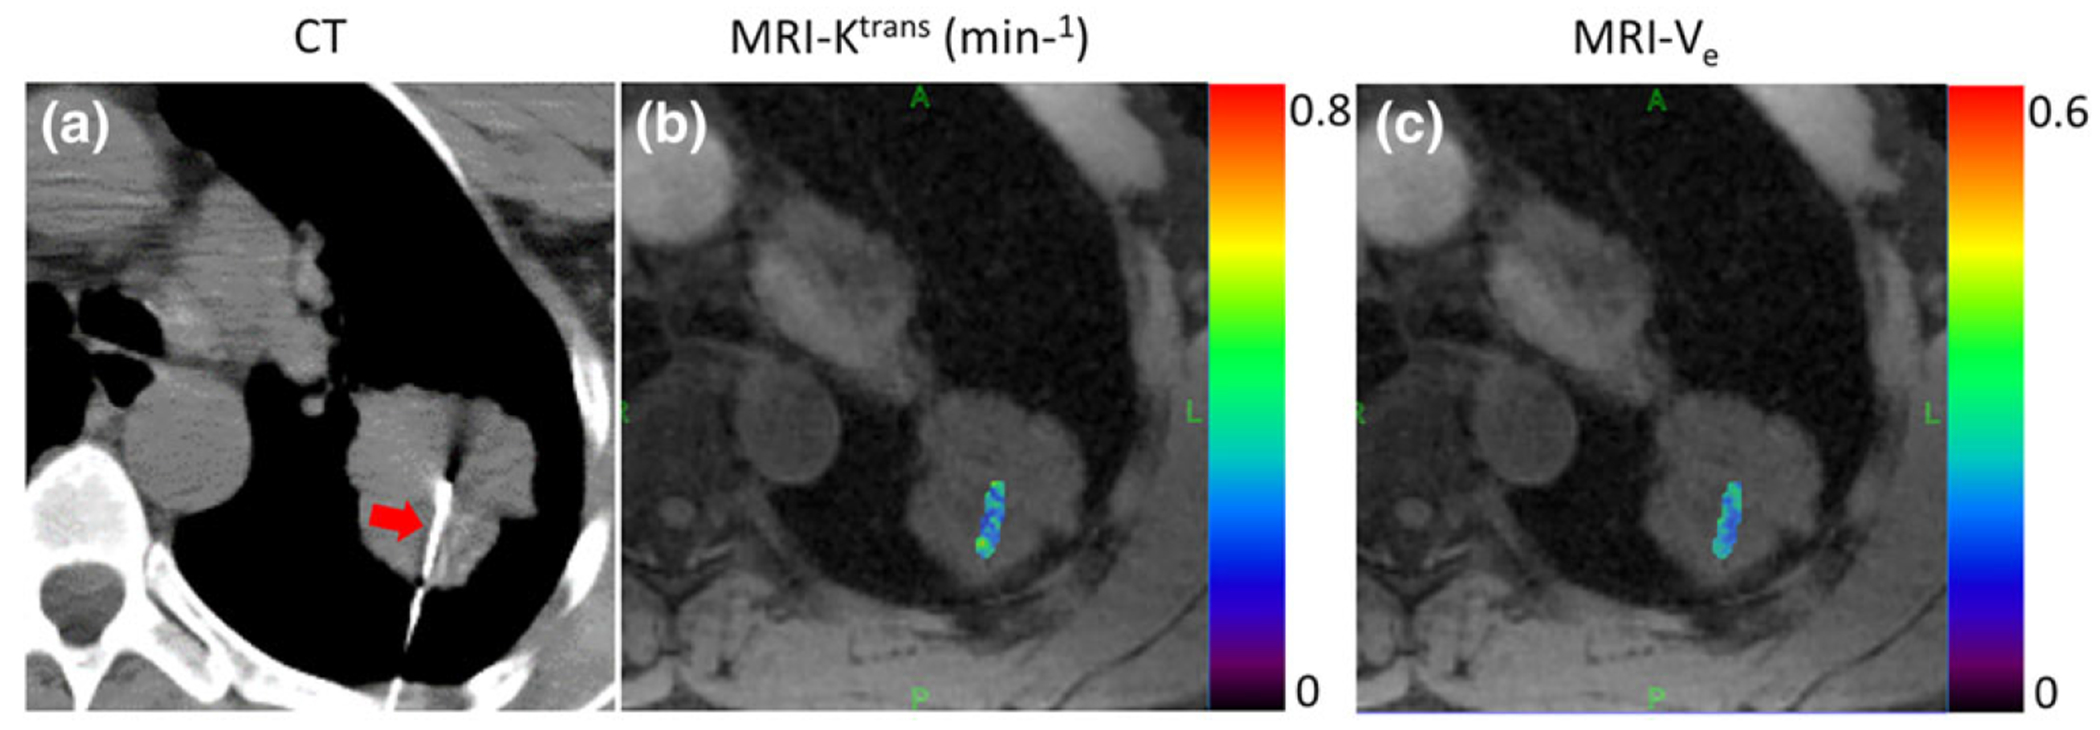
Regional K^trans^ and V_e_ maps (b,c) obtained from a patient with squamous cell carcinoma superimposed on a corresponding GRASP image. The ROI for perfusion analysis was selected using CT-biopsy image (a) as a reference, as previously shown in Fig. 2. The red arrow indicates the needle in CT biopsy.
